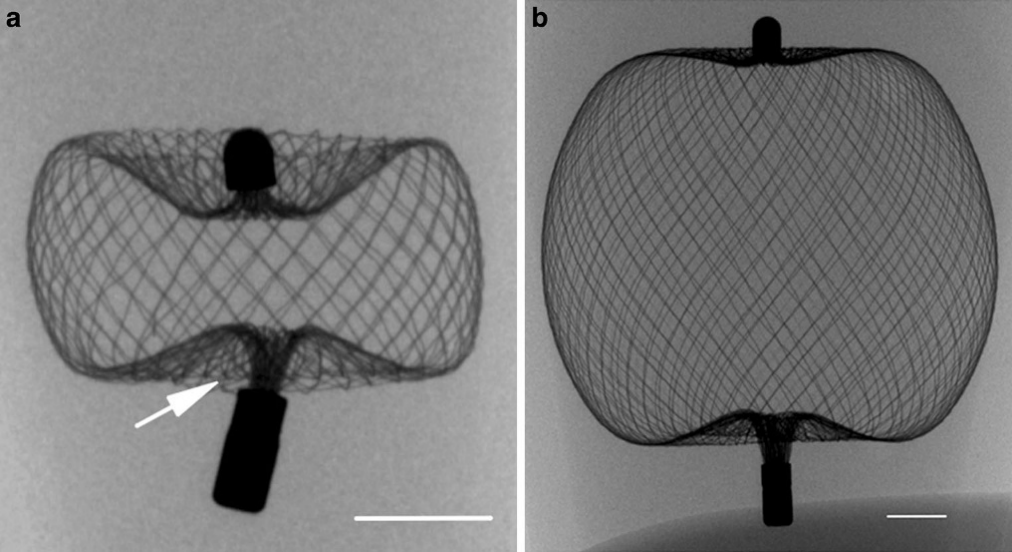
Microcomputed tomography (CT) fluoroscopy of the rescued WEB devices SL 3.5 × 2 mm (a) and SLS 8 (b). The white arrow indicates the slight deformation at the proximal marker. Scale bar = 1 mm
